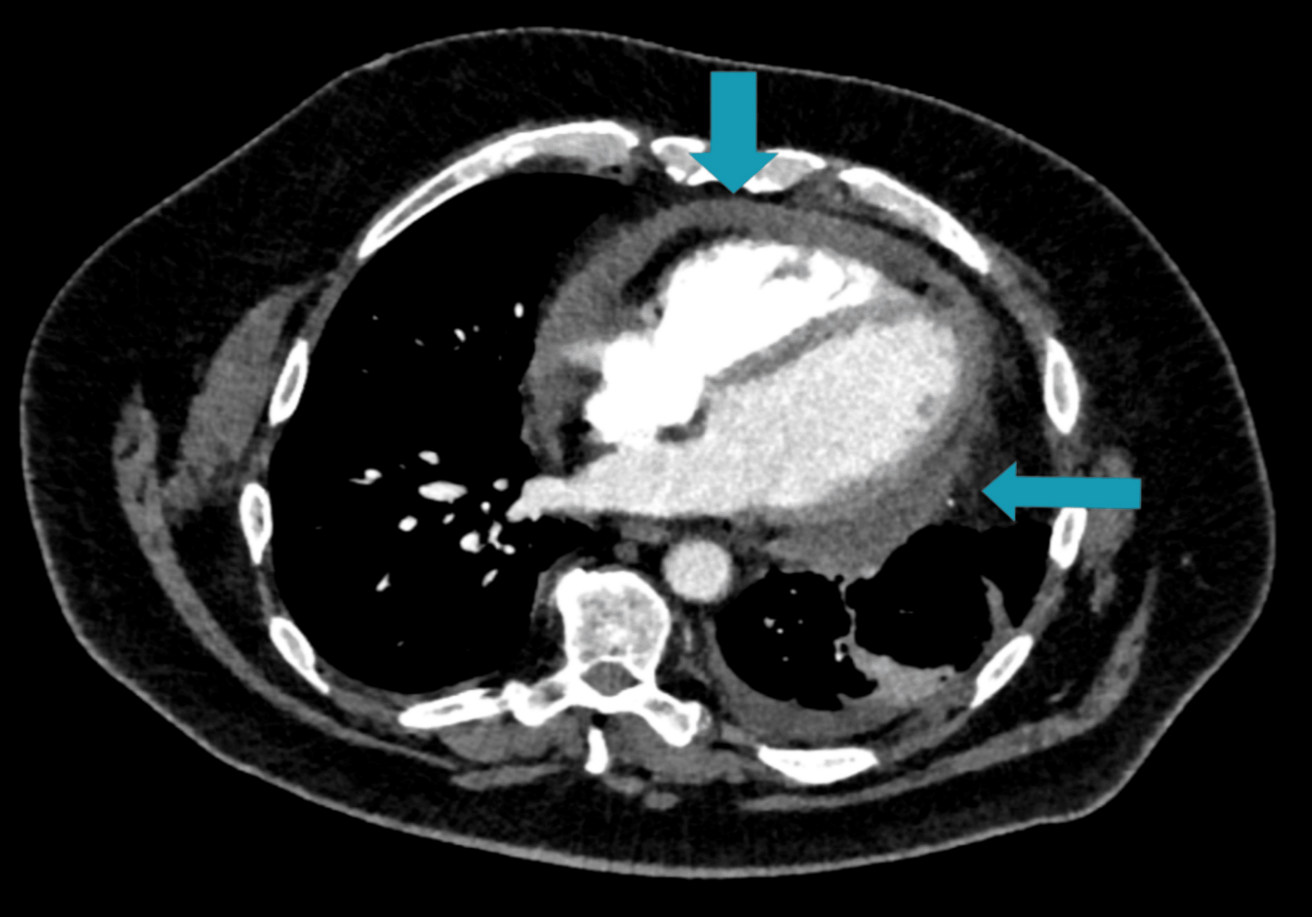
Contrast-enhanced axial CT chest demonstrating a moderate pericardial effusion (hypodense rim surrounding the heart) with associated inflammatory stranding in the adjacent pericardial fat and mediastinal lymphadenopathy, raising the possibility of pericarditis.
